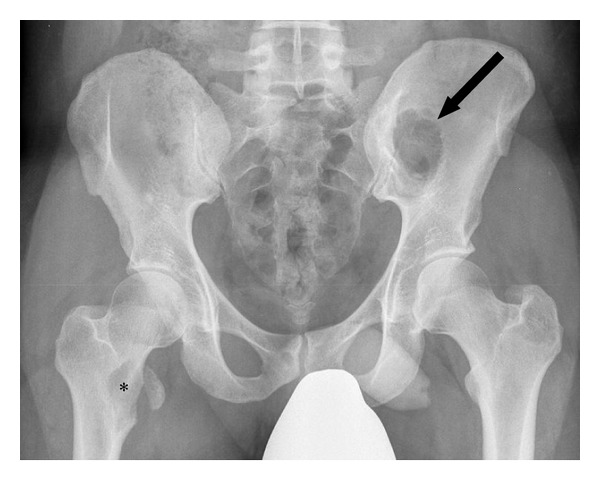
Conventional radiography, AP view of the pelvis: lytic lesions of the right proximal femur (asterisk) and of the left ileum (arrow) became obvious.
